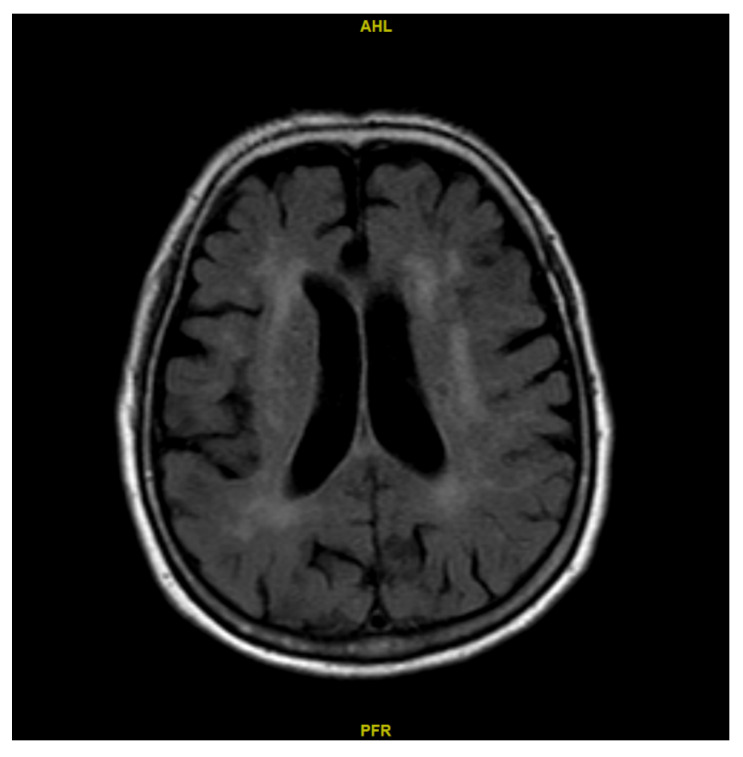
Axial MRI (T2-weighted FLAIR) showing left–right ventricular asymmetry and mild periventricular white matter hyperintensity.
